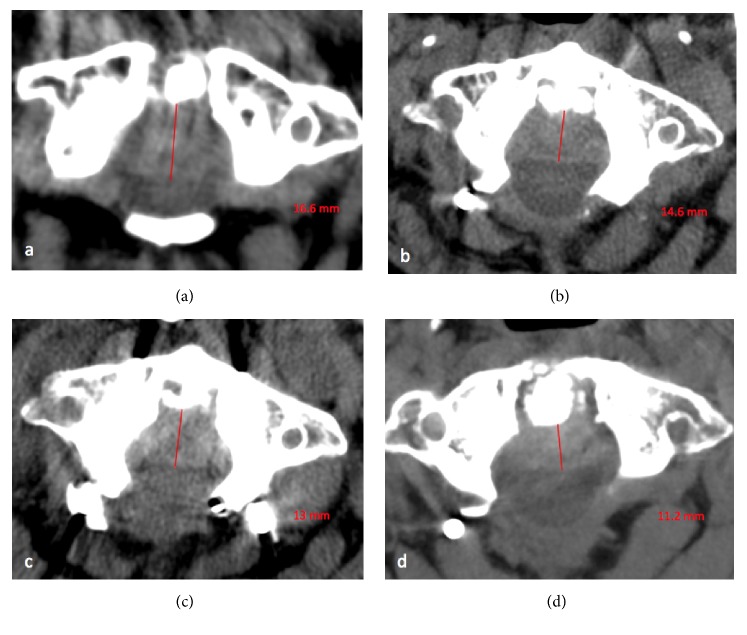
Comparison of the size of ROP on preoperative CT and postoperative CT with soft tissue window and in axial plane. We see the regression of size of the ROP between preoperative CT (a) with thickness of 16.6 mm under the os odontoideum and the postoperative CT just after surgical management (b) with thickness of 14.6 mm, at 4 months with thickness of 13 mm and at 6 months with thickness of 11.2 mm after surgery.
